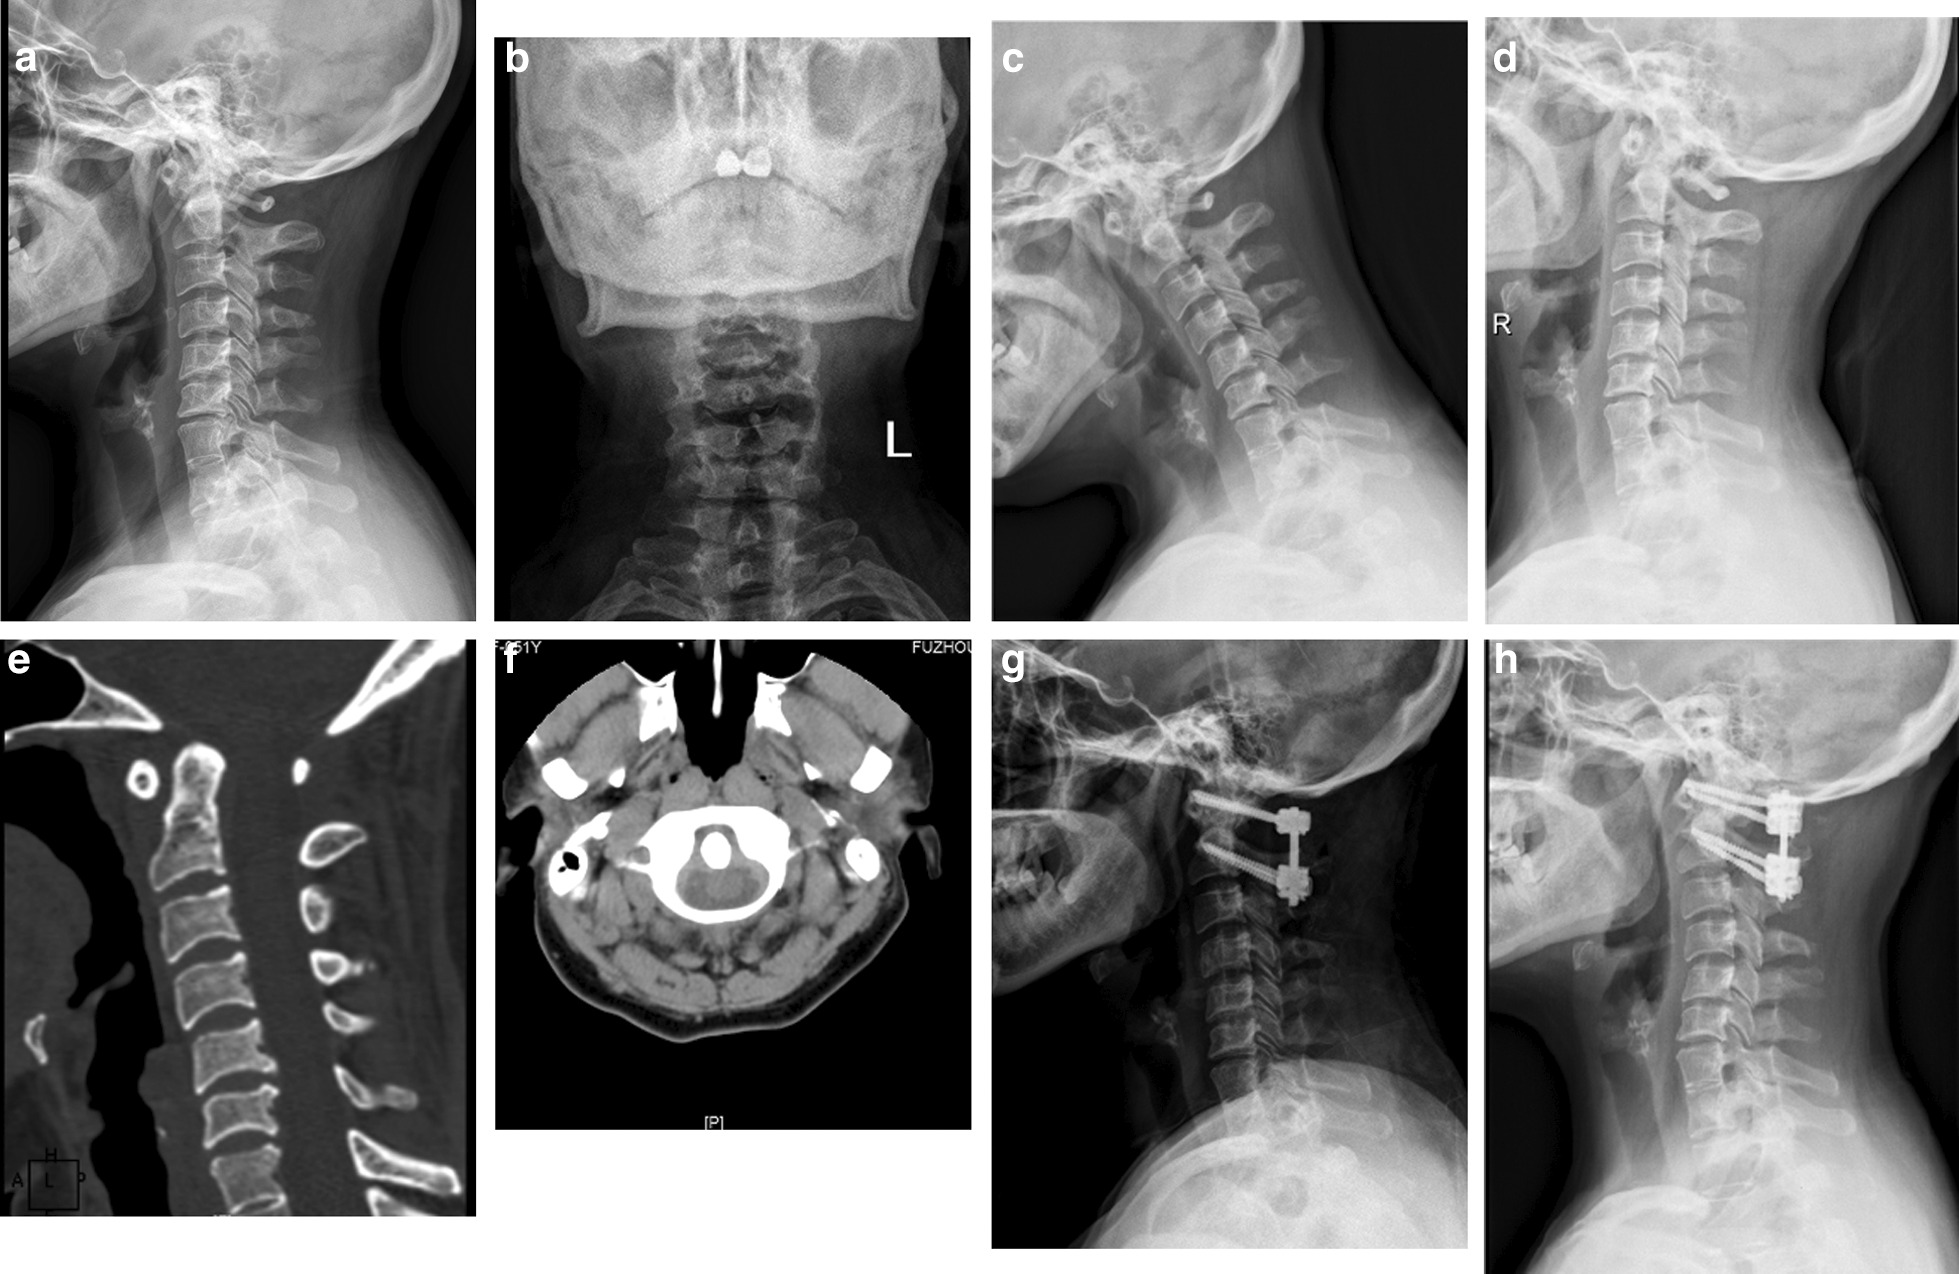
Atlantoaxial dislocation (Type II), female, 51 years old. a, b Lateral and open-mouth view radiographs of cervical spine showed that the C2–C7 angle was 8°. c, d Flexion–extension radiographs of cervical spine indicated a partially reducible dislocation of the atlantoaxial joint. e, f CT scan of cervical spine also indicated the atlantoaxial dislocation. g One month after operation, lateral radiograph of cervical spine showed that the dislocation had been fully reduced, and the C2–C7 angle was 8°. h Seven months after operation, lateral radiograph of cervical spine showed the loss of subaxial lordosis and the C2–C7 angle was 2°
